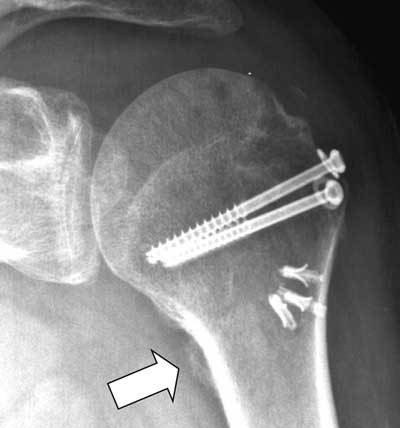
Radiograph showing ossification (arrow) at the insertion side of inferior glenohumeral ligament appeared after antero-inferior dislocation of the shoulder.
